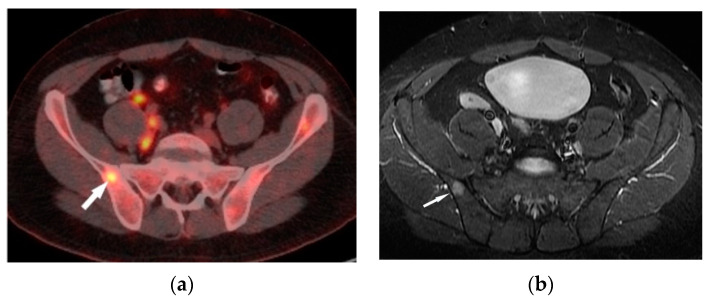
FDG-PET/CT (a) and T1–weighted MRI image with contrast enhancement (b) presents an enhanced lesion with increased FDG uptake (white arrows) in the right iliac bone.
